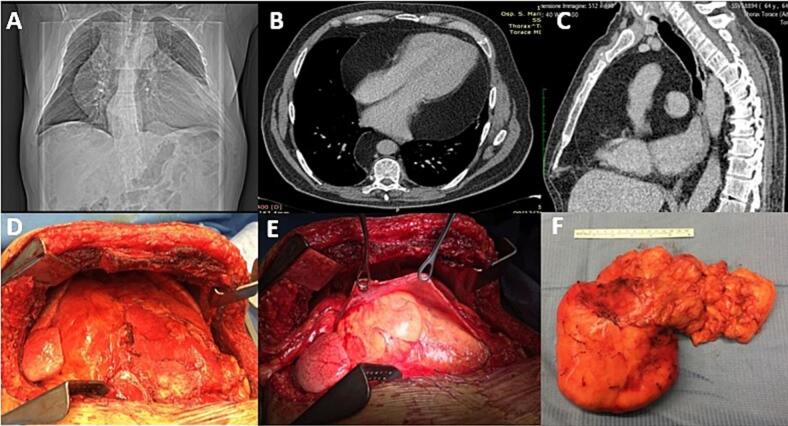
A: Chest Rx; B: thorax CT scan; C: sagittal reconstruction of thorax CT scan; D: view of the lesion after clamshell incision; E: view of the lesion after pericardial incision; F: large lipoid mass extracted from pericardium.
